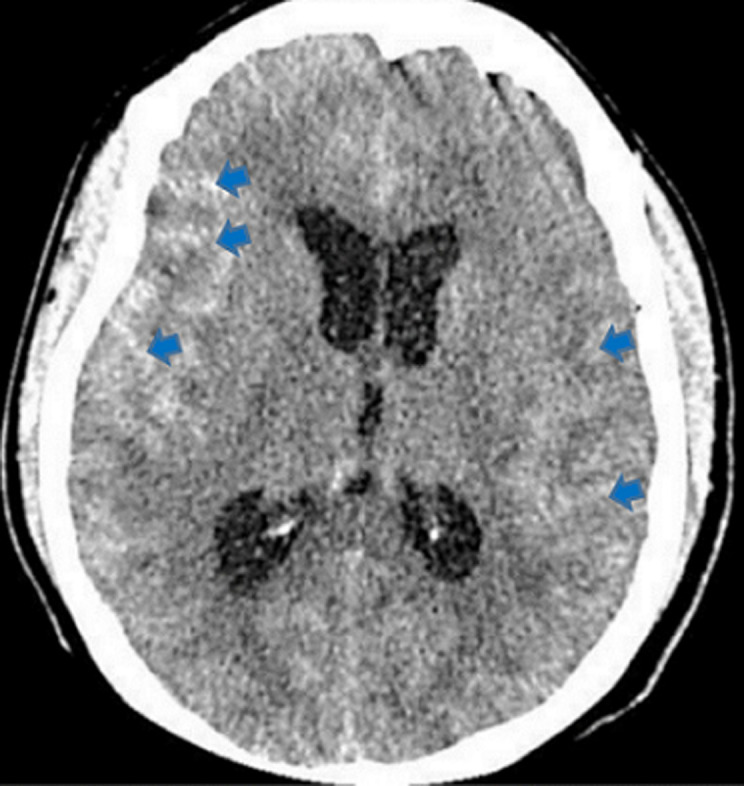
an axial non-enhanced CT scan of the head showing hyperdense collections of acute blood attenuation in the sulci in keeping with acute subarachnoid hemorrhage (blue short arrows)
